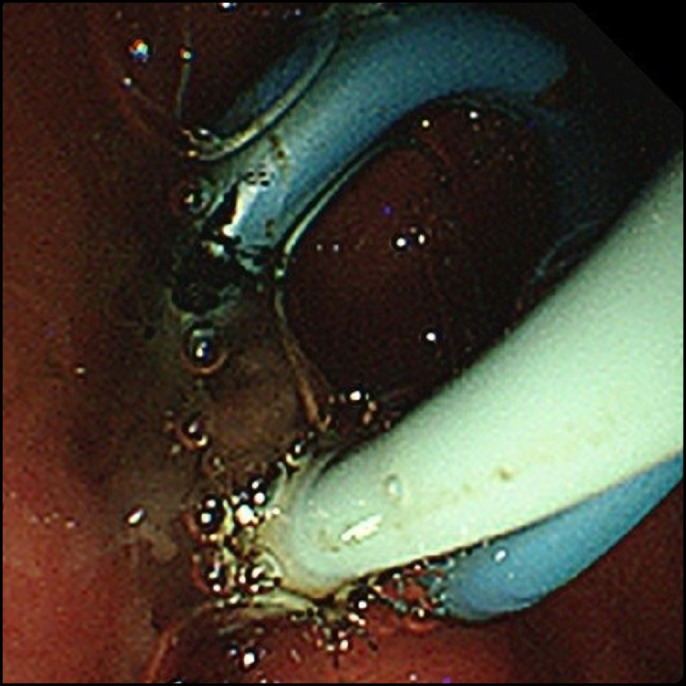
Endoscopic ultrasound-guided pancreatic pseudocyst drainage, with successful internal and external drainages.
